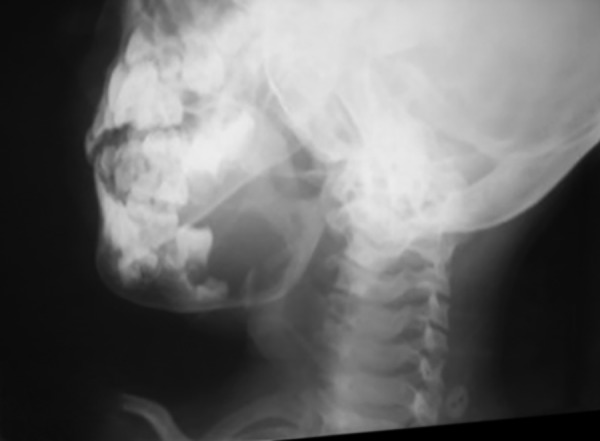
The lateral oblique radiograph of the jaw
